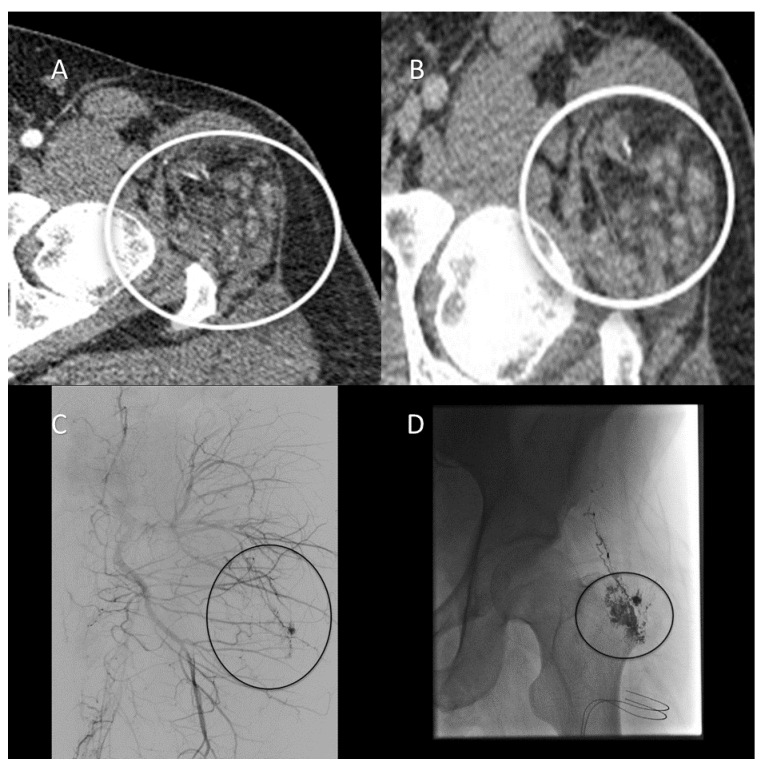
A 37-year-old man with Cowden syndrome and follicular thyroid cancer with a history of left hip pain and palpable abnormality. (A) Arterial-phase computed tomographic (CT) angiography of the lower extremities demonstrates an arterially enhancing lesion containing abnormal vasculature involving the left inferior gluteus medius muscle adjacent to the left hip supplied by branches of the lateral circumflex femoral artery (circle). (B) Venous-phase CT angiography demonstrates persistent enhancement consistent with an arteriovenous malformation (AVM). (C) Pre-embolization digital subtraction angiogram demonstrates an AVM supplied by branches of the left superior gluteal and lateral circumflex femoral arteries (circle). (D) Postglue embolization angiogram demonstrates stasis within AVM (circle).
